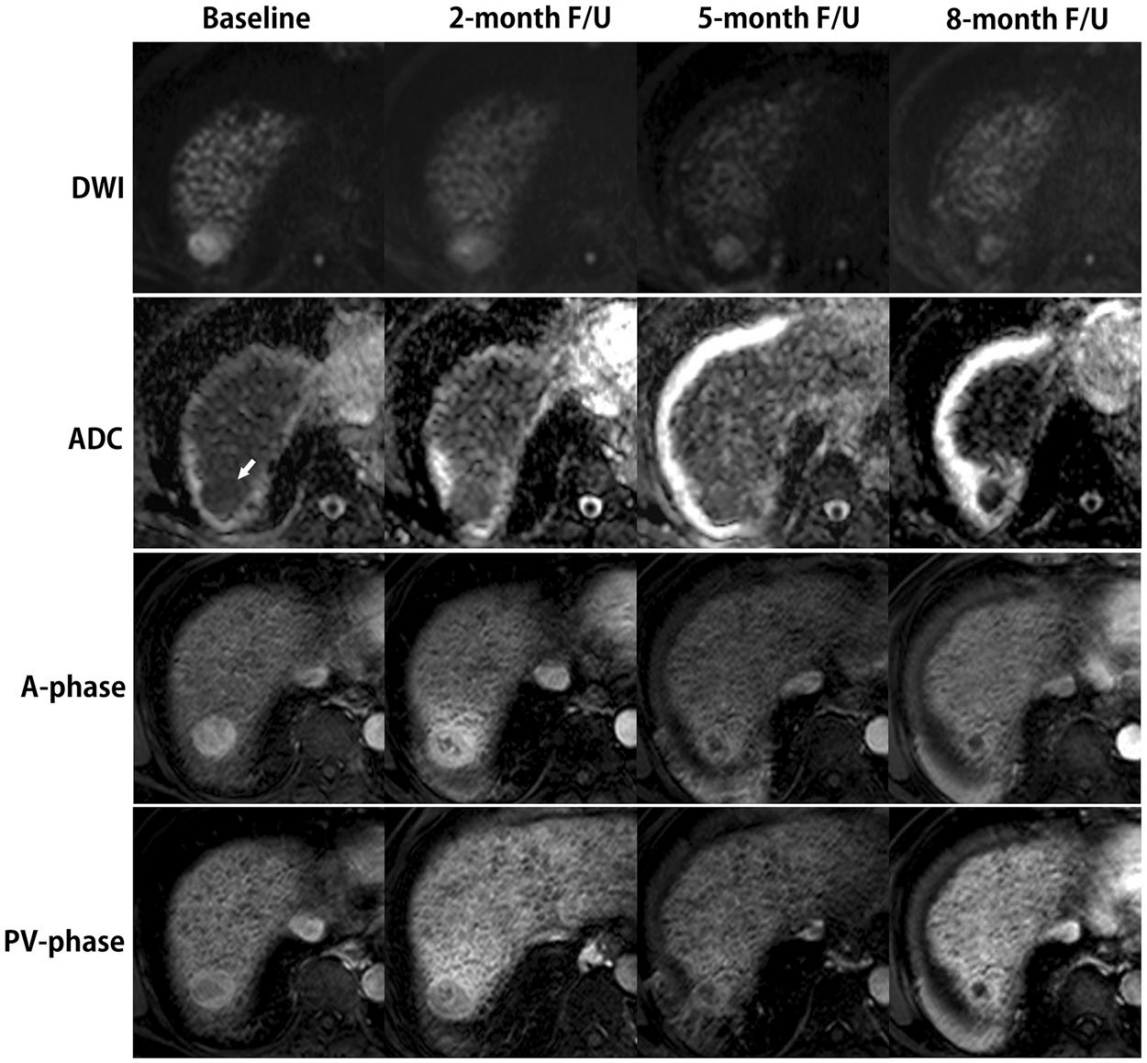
Early ADC change before HCC lesion reduction obtained at baseline and at different times post-SABR. A 71-year-old man with hepatitis B virus-related liver cirrhosis and a 2.8 cm HCC in segment 7. At baseline MRI, the lesion shows strong enhancement in the arterial phase (A-phase) and washout in the portal venous phase (PV-phase), with moderate hyperintensity on DWI (*b* = 500) and an ADC of 1.37 × 10^−3^ mm^2^/s. At the 2-month follow-up, the lesion was stable in size by mRECIST. Lower hyperintensity at DWI was noted with a corresponding ADC of 2.00 × 10^−3^ mm^2^/s (a 46% increase). Geographic parenchymal hyperenhancement was noted in both the A- and PV-phases, consistent with focal liver reaction to SABR. At the 5-month follow-up, the lesion had a lower enhancement size (1.9 cm), indicative of partial response, with resolution of geographic parenchyma hyperenhancement. At the 8-month follow-up, no enhancement was observed, indicating complete response. Continuous volume loss of the overlying liver parenchyma was observed at 5 and 8 months.
